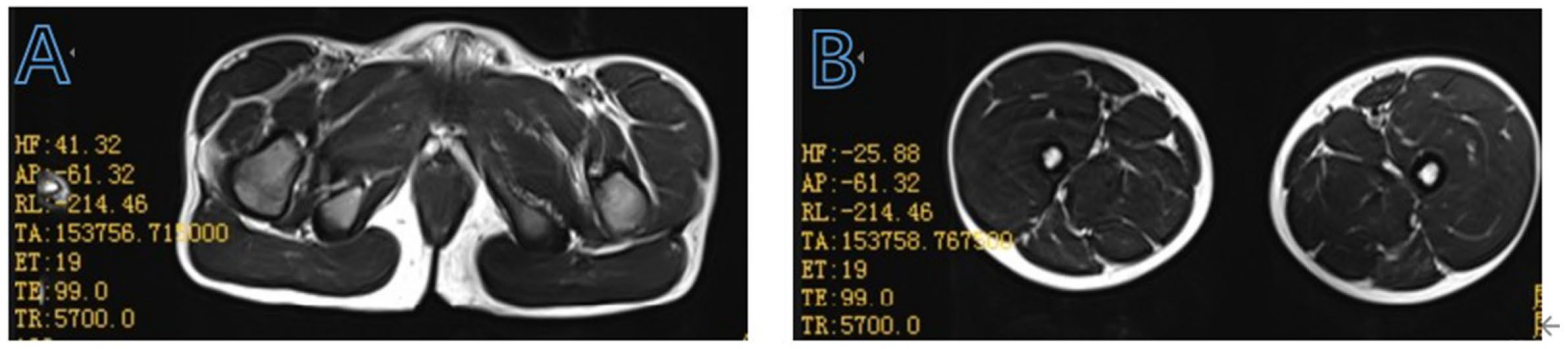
Muscle magnetic resonance T2-weighted imaging features of the enrolled patient at the pelvis (A), bilateral thigh (B).
